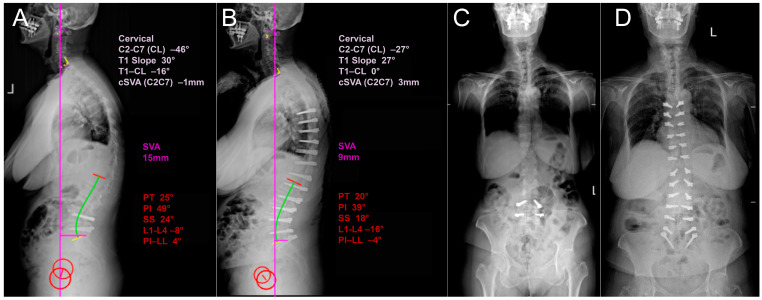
Dynamic X-rays of a patient with sagittal deformity. (A) Pre-operative and (B) post-operative sagittal dynamic X-rays displaying parameters of the spine. (C) Pre-operative and (D) post-operative dynamic coronal X-rays.
